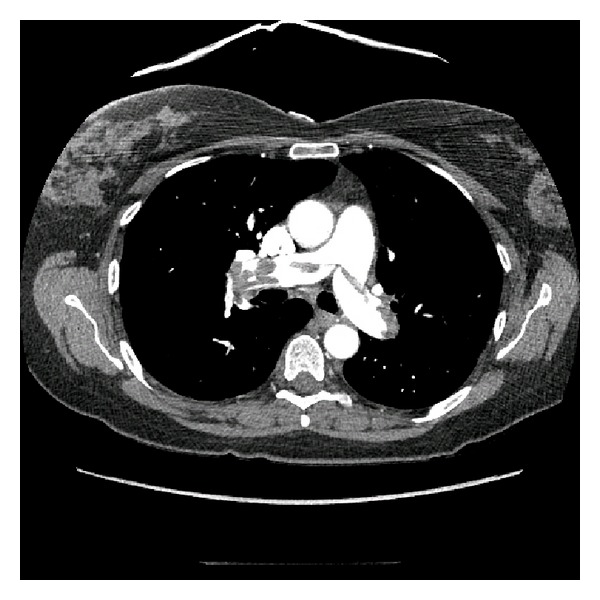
CT angiogram of the chest demonstrating bilateral pulmonary embolus.
